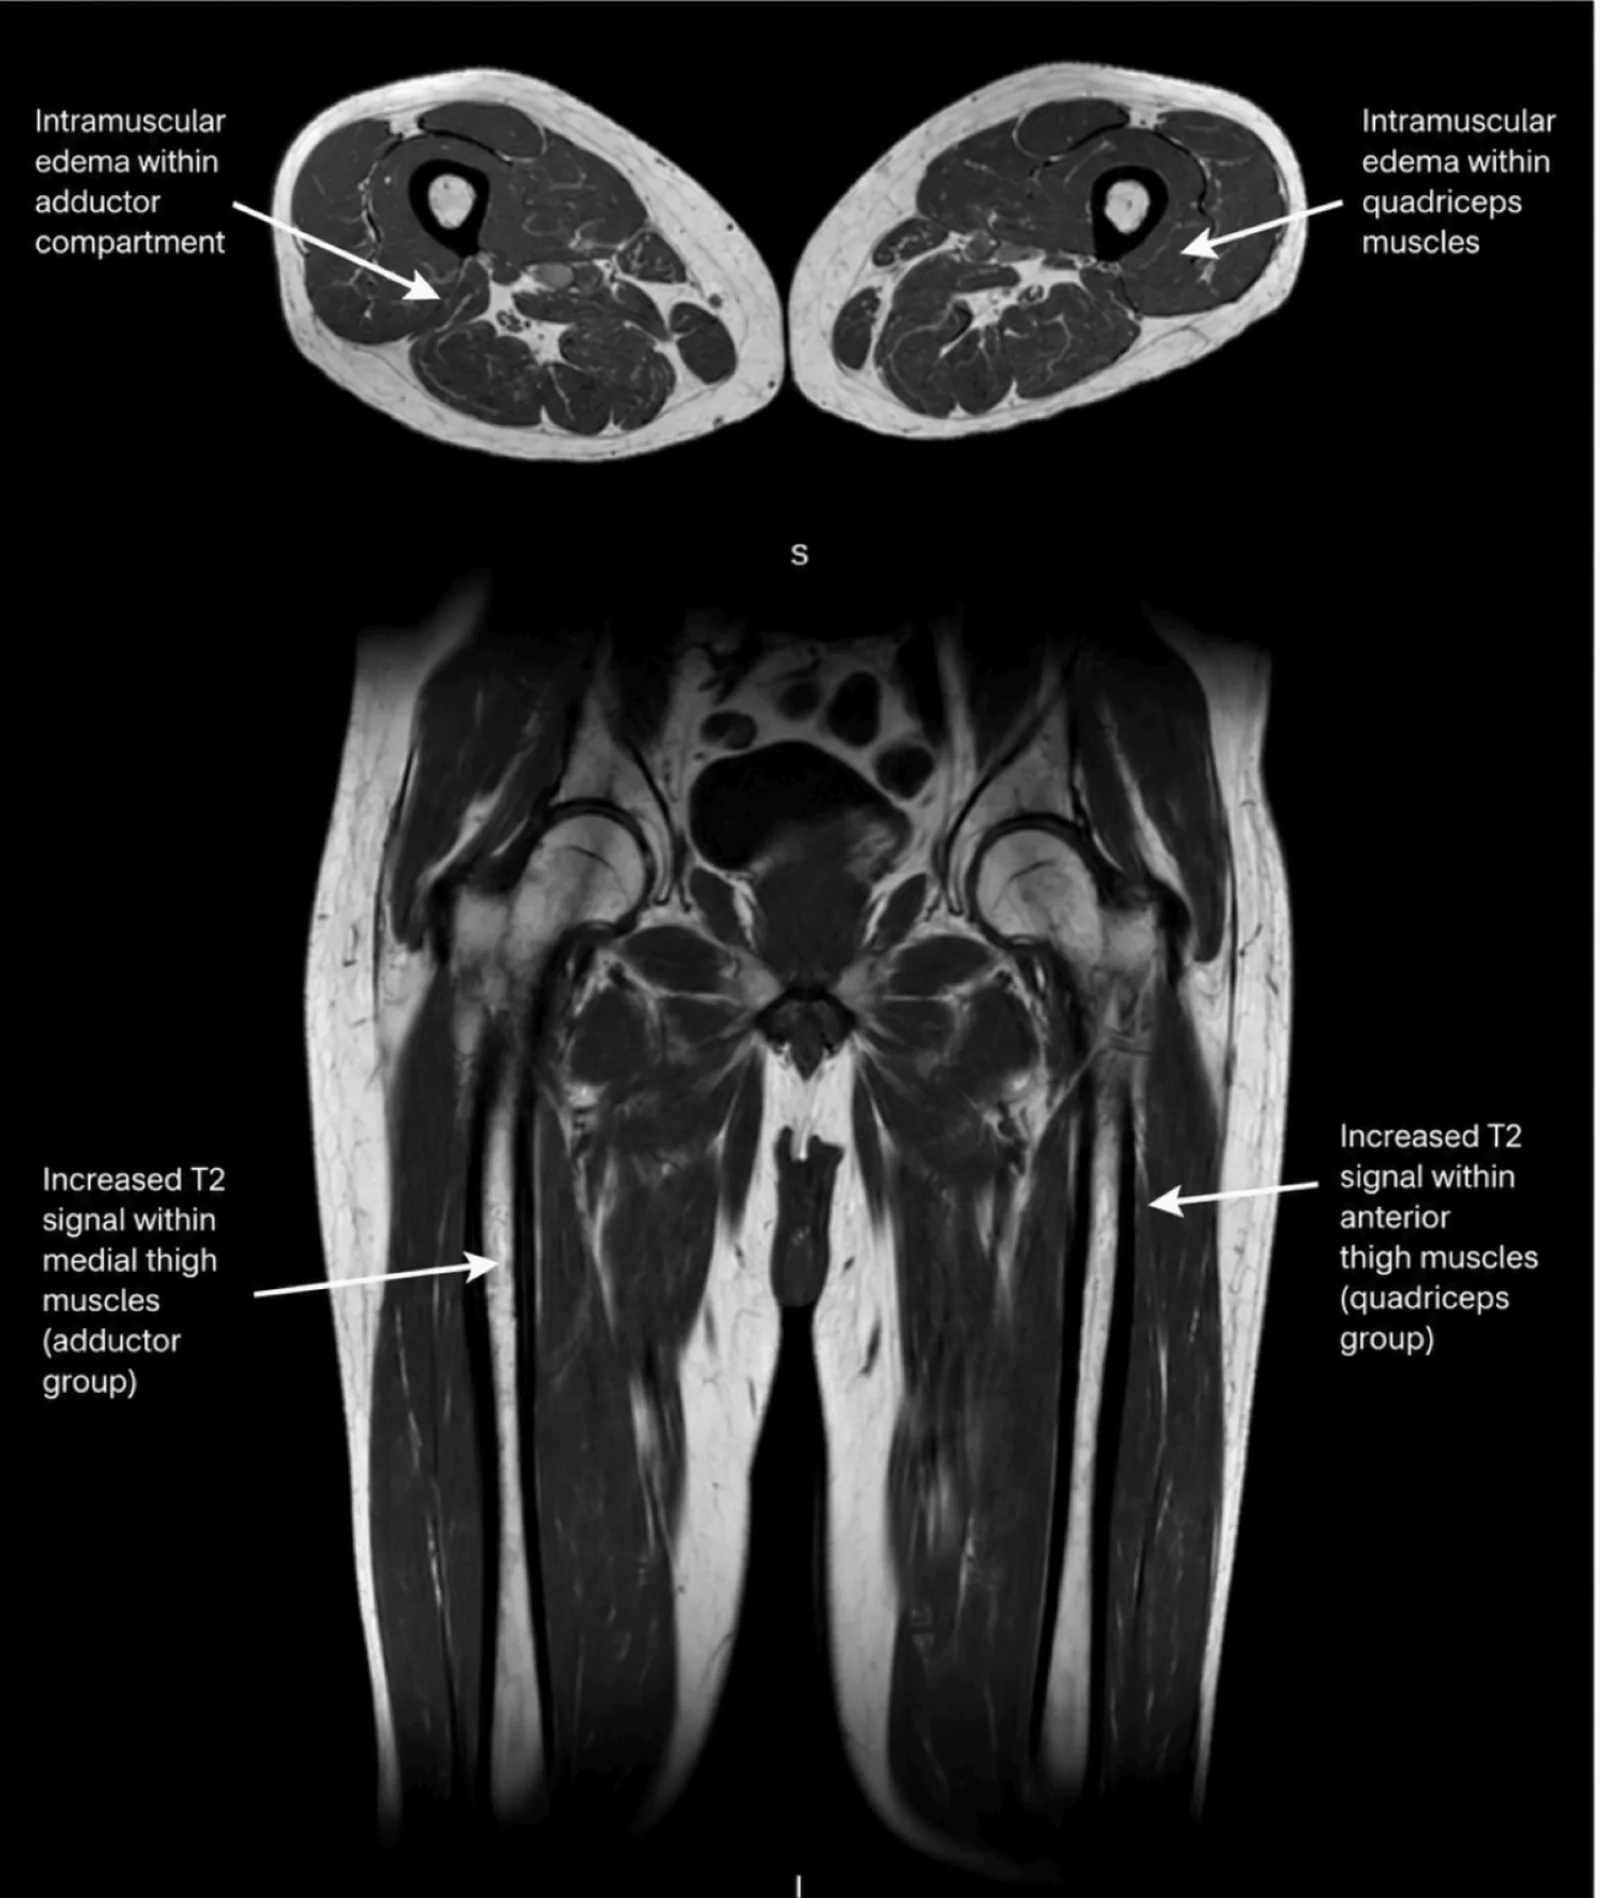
MRI of the thighs demonstrating bilateral posterior paraspinal muscle oedema with trace fluid tracking along the iliopsoas muscles (greater on the right) and small hip and knee joint effusions, consistent with inflammatory myositis and inflammatory arthritis MRI: magnetic resonance imaging
